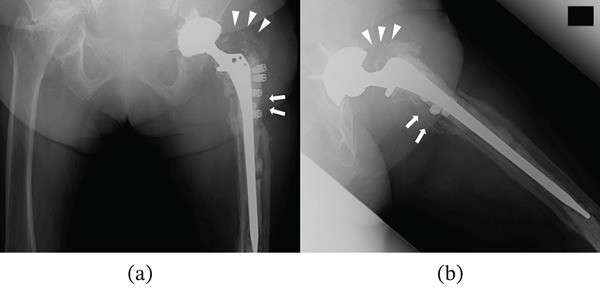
Radiographs at the initial visit: (a) anteroposterior view and (b) lateral view. Extensive osteolysis is seen in the proximal lateral region of the femur, where the infection has spread (arrowheads). The wires are loosened and have been dislocated from their original positions (arrow).
